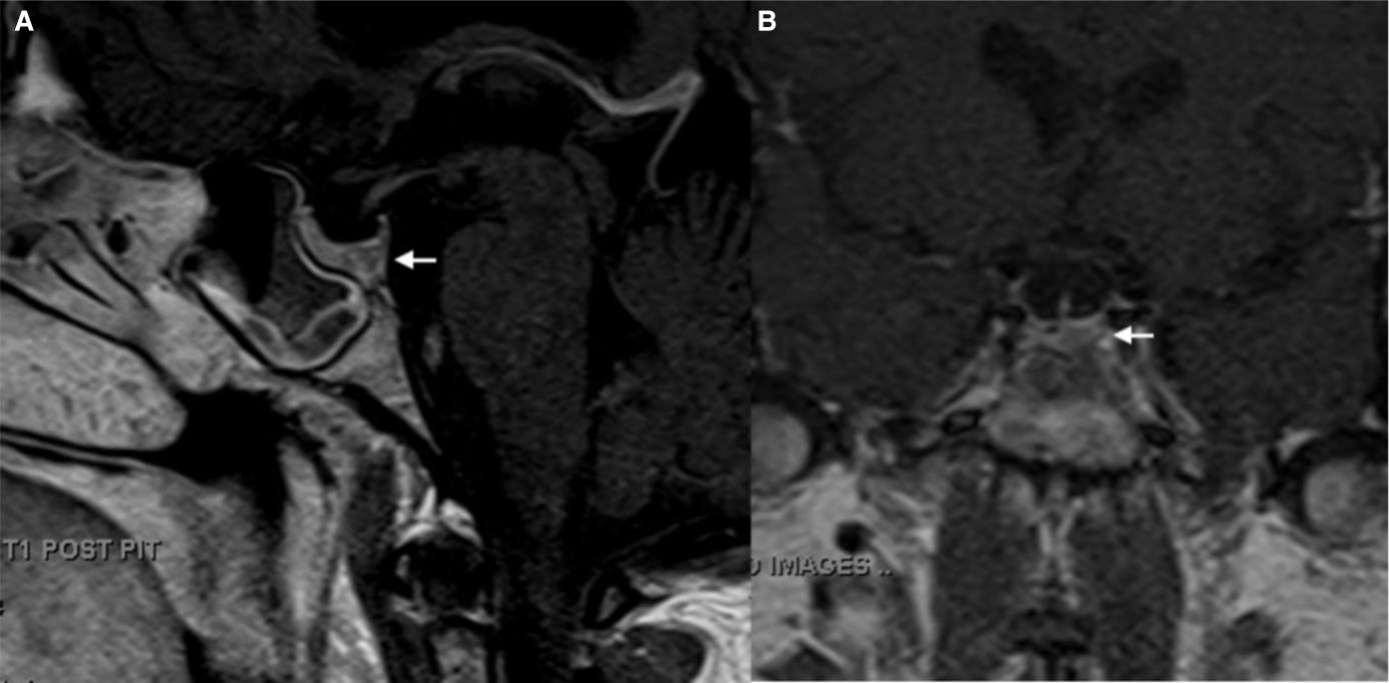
T1-weighted magnetic resonance imaging of the brain (MRI) after intravenous Gadolinium contrast. (A) Sagittal view of the MRI revealed a hypo-enhancing lesion in the pituitary gland, sized 5.3 × 3.3 mm. (B) Coronal view of the MRI revealed a hypo-enhancing lesion on the left side of the sella turcica, causing the asymmetrical appearance. The sphenoid sinus was noticed to be opacified in the MRI.
